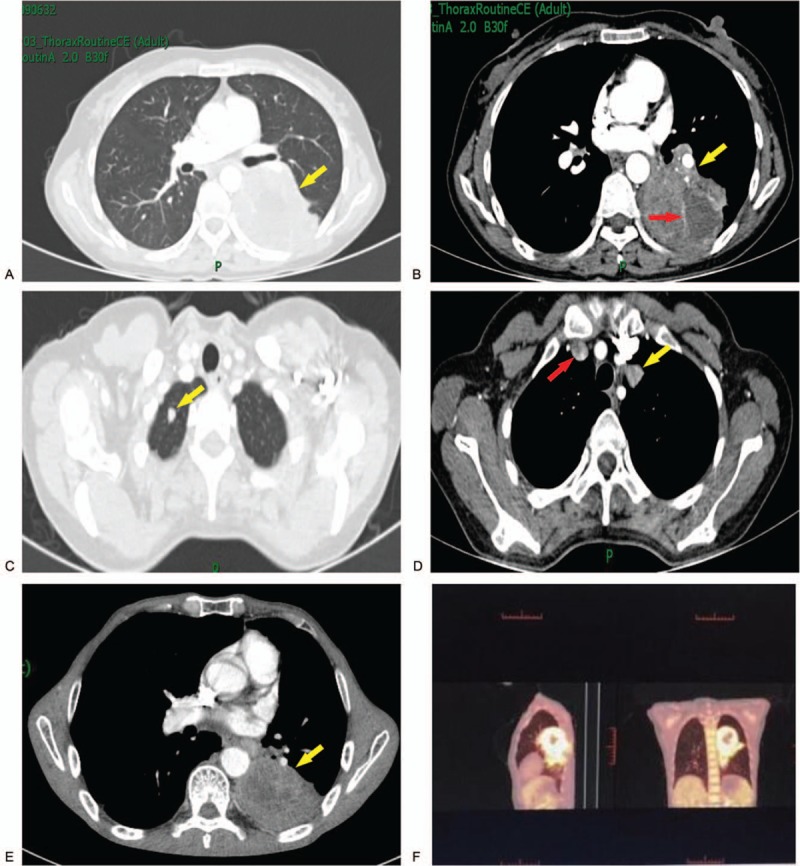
Comprehensive enhancement CT scan results. (A) A well-circumscribed soft tissue mass in the posterior segment of the left lung near the hilum (yellow arrow). (B) A low-density necrotic lesion (red arrow) in the tumor (yellow arrow) and an inhomogeneous enhancement. (C) Soft tissue in the apex of the right lung (yellow arrow). (D) Soft tissue in the left upper lobe (yellow arrow) and swollen lymph nodes in the mediastinum (red arrow). (E) The tumor size significantly shortened the posterior segment of the left lung (yellow arrow). (F) The mass near the left hilum shows high fluorodeoxyglucose (F^18^-FDG) uptake (SUVmax, 10.72).
